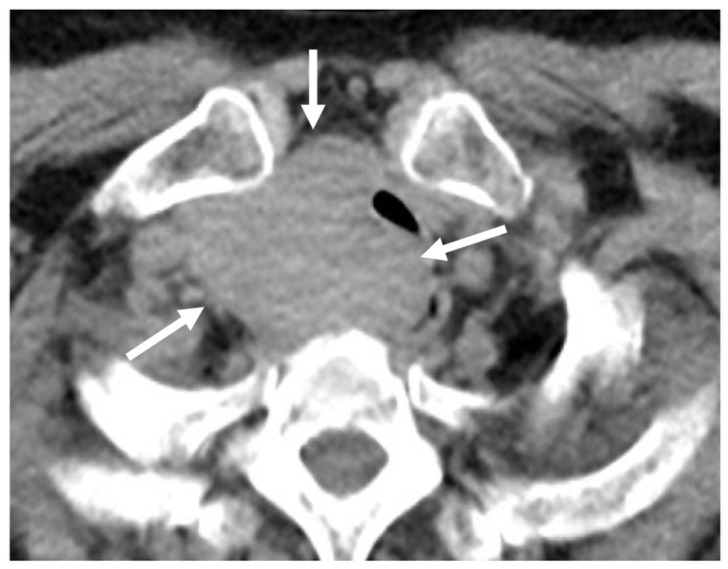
Incidental thyroid mass in low-dose computed tomograph (LDCT). Axial non-contrast LDCT of 66-year-old female demonstrates large, homogeneous right thyroid mass (arrows) with mass effect and marked narrowing of tracheal lumen.
